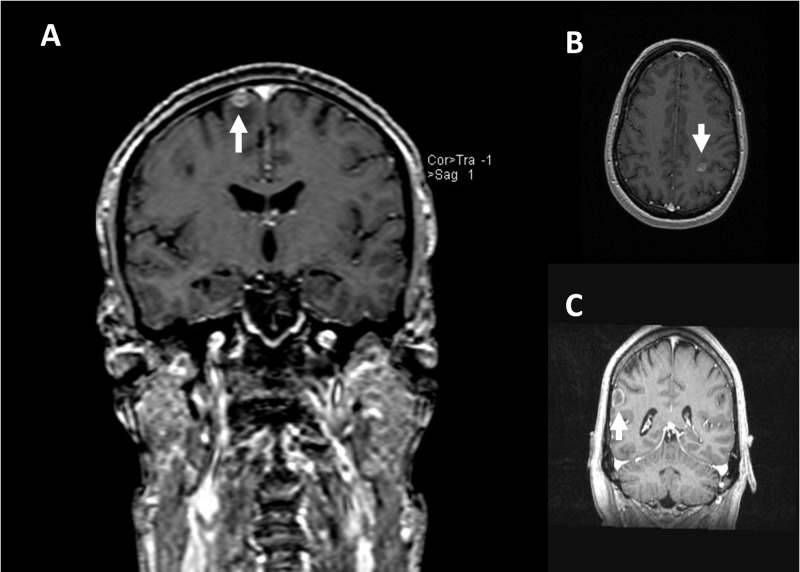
Patient 1 magnetic resonance imaging (MRI) causing division between readers T1-weighted gadolinium enhanced images of candidate lesions causing division between readers (A: Coronal view, B: Axial view, C: Coronal view).
